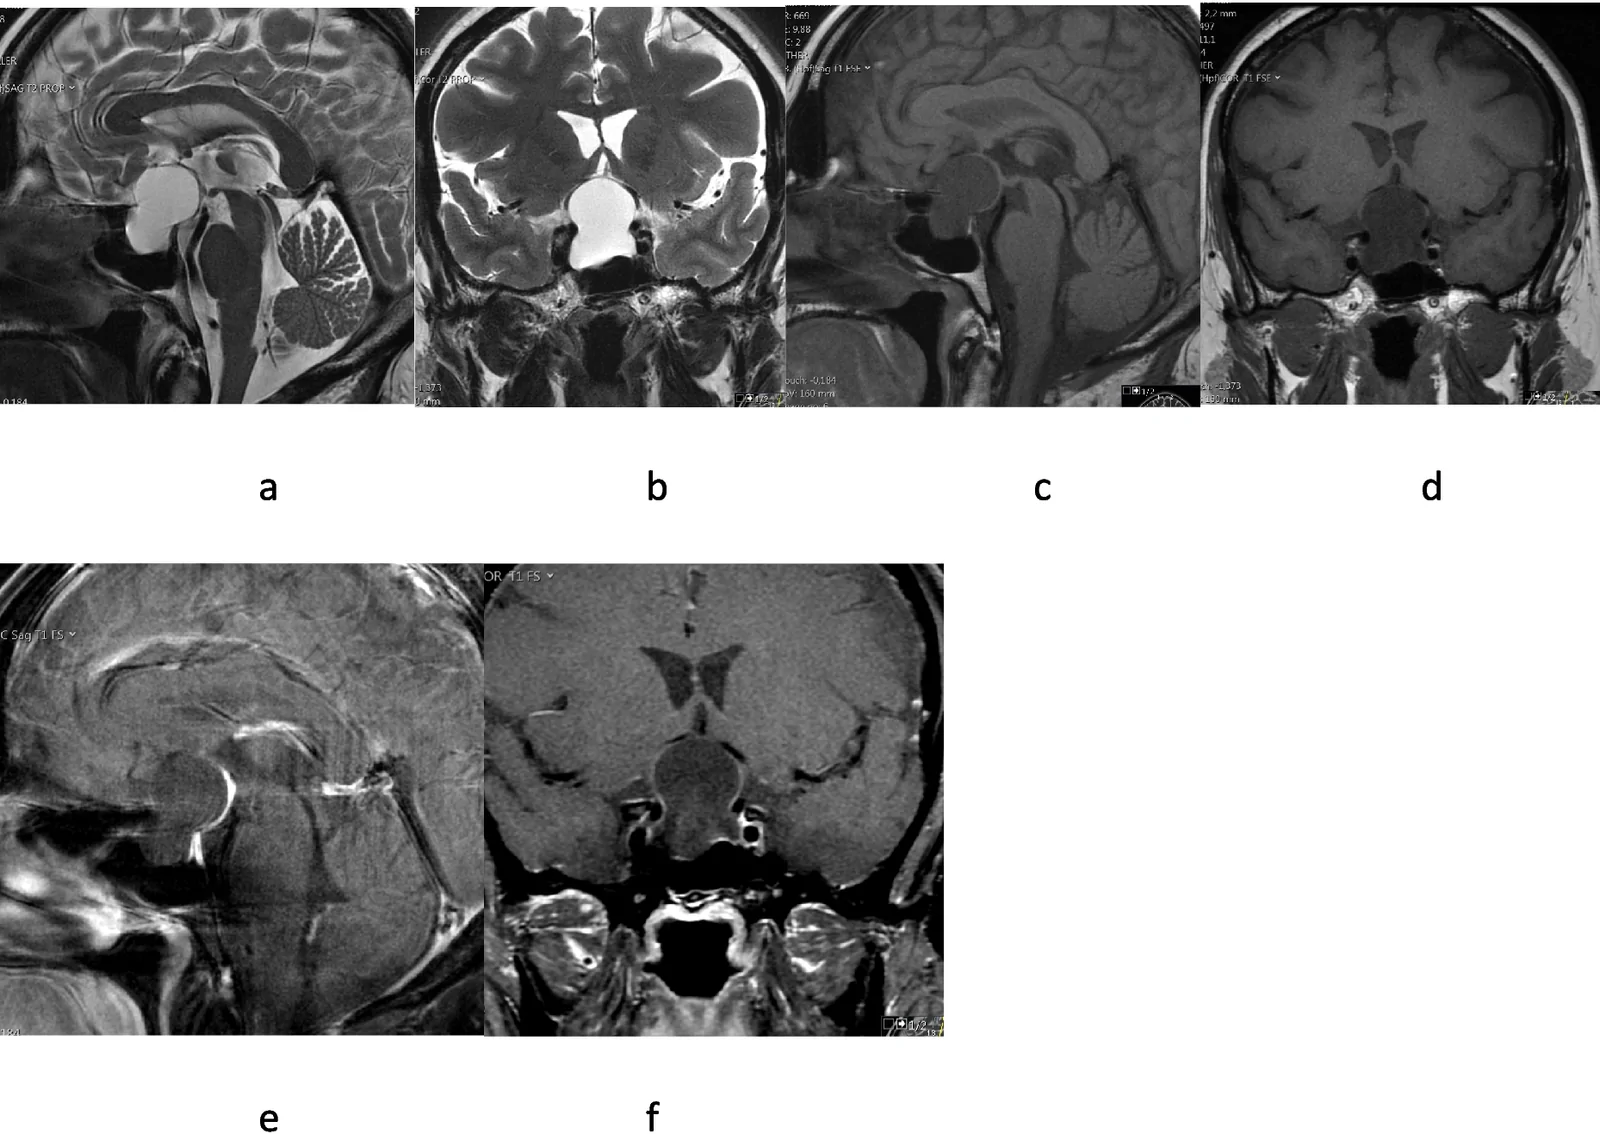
The case of a 46-year-old female with a surgically confirmed arachnoid cyst. Sagittal and coronal planes of T2- and T1-weighted images (a-d) reveal a large and well-delineated sellar lesion with a significant suprasellar extension. The signal intensity of the lesion is similar to that of CSF. The lesion has a thin wall with no enhancement on the sagittal and coronal contrast-enhanced T1-weighted images (e, f). Note that the pituitary gland and stalk appear flattened and stretched over the posterior boundary of the cyst
